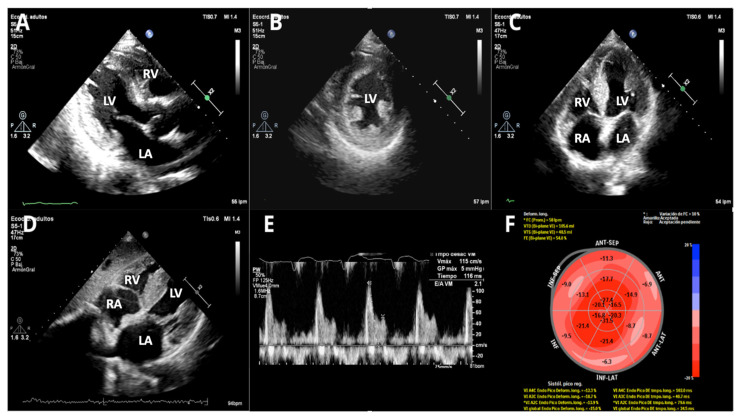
Transthoracic echocardiography (TTE). (A) Parasternal long axis—2D, (B) parasternal short axis—2D, (C) apical 4-chamber—2D, (D) subxiphoid long axis—2D, (E) Doppler Pulsatile wave-restrictive mitral flow, and (F) bull eyes, speckle tracking 2D apical myocardial sparing. It shows concentric left ventricular hypertrophy with normal left ventricular ejection fraction and grade III diastolic dysfunction. There is also right ventricular free wall thickening and moderate biatrial enlargement with interatrial septum thickening. (LA: left atrium, LV: left ventricle, RA: right atrium, RV: right ventricle).
